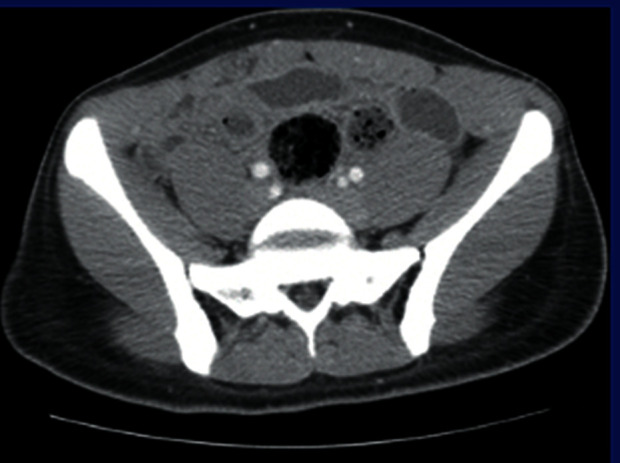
CT of the abdomen and pelvis with contrast showing distended loops of small bowel with wall thickening, enhancement, decompressed loops of bowel distally, and fecalization of small bowel.
